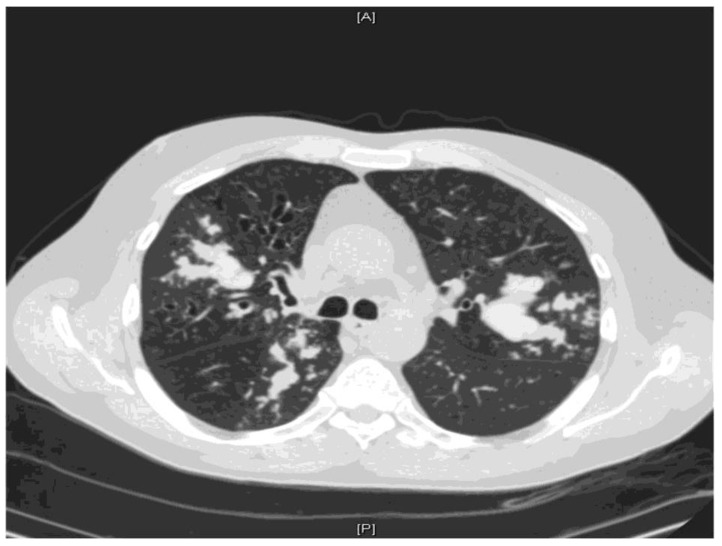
CT thorax showing severe multilobar bronchiectasis with accumulation of high-attenuation mucus forming bronchoceles predominantly within the right upper lobe, the right lower lobe and the left upper lobe.
